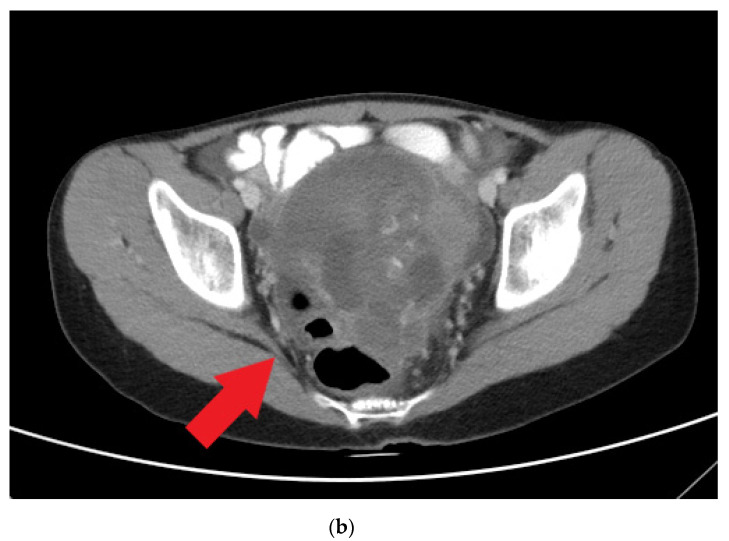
(a) Heterogeneous adnexal mass confirmed by sonography (red arrow). (b) Computed tomography scan revealing a lobulated mass lesion (maximum diameter of 10.1 cm) with cystic and solid components in the pelvis (red arrow).
